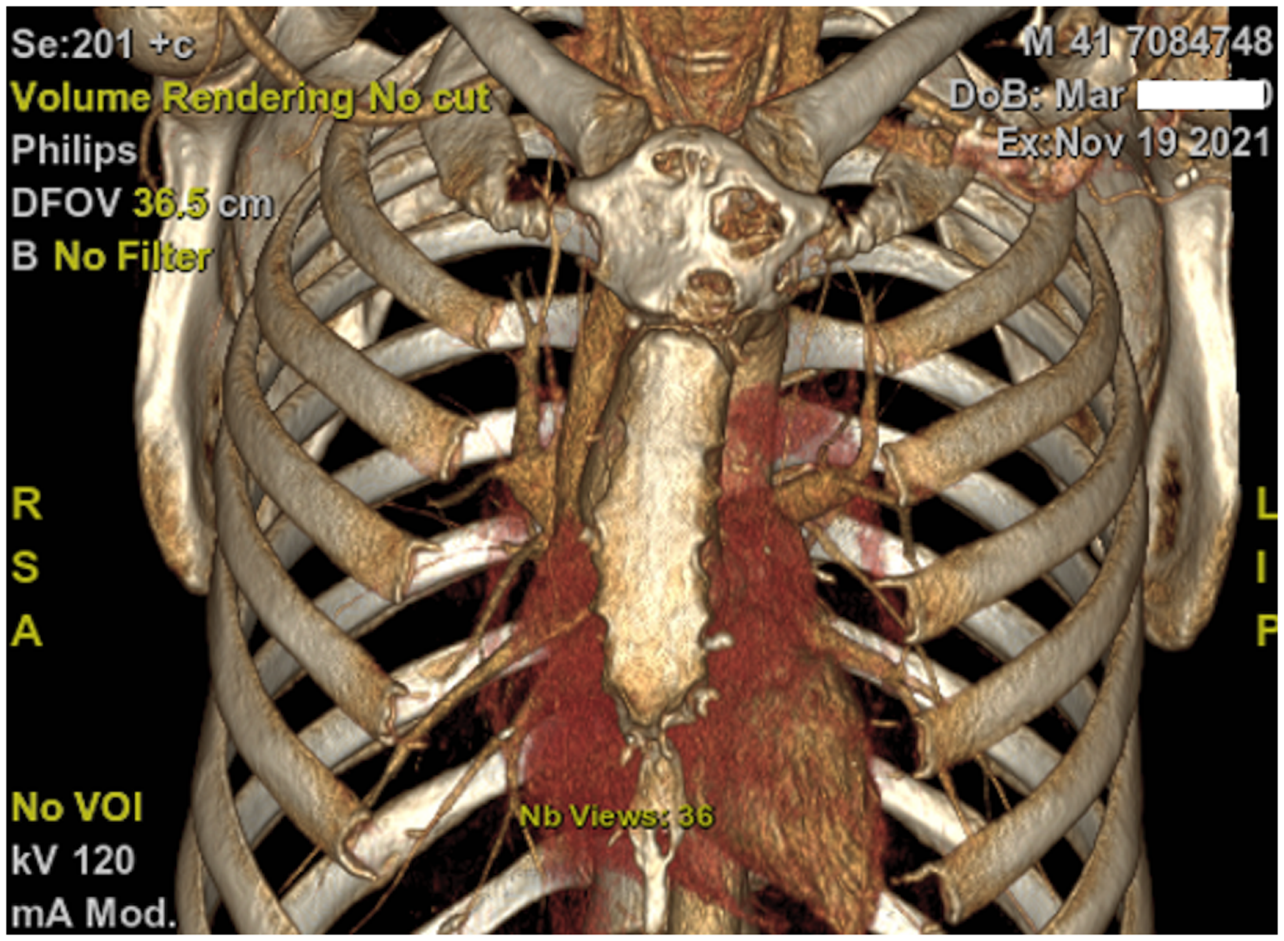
Computed tomography with three-dimensional reconstruction revealed a heterogeneous, cystic bony lesion limited to the manubrium sterni.
